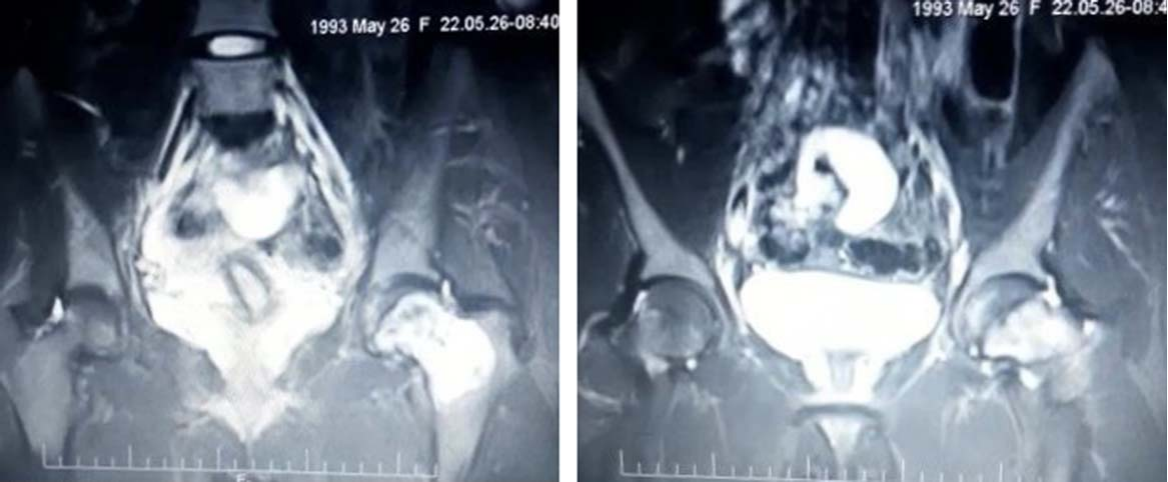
T1 weighted MRI showing osteolytic lesion with breach in the cortex.
